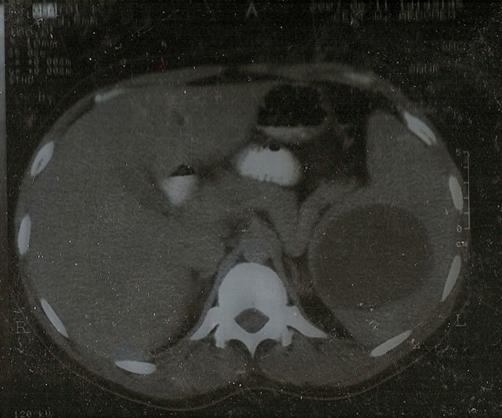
CT abdomen showing splenic hypointense homogenous lesion.
